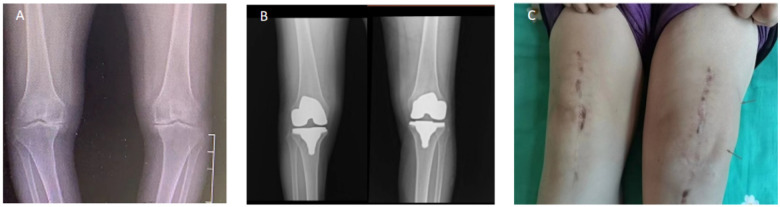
Radiographic and clinical presentation of the patient before and after total knee arthroplasty. (A) Preoperative standing anteroposterior radiograph showing severe bilateral knee osteoarthritis with varus deformity. (B) Postoperative radiograph after bilateral total knee arthroplasty demonstrating appropriate prosthesis alignment and positioning. (C) Clinical image showing significant swelling of the left knee approximately 11 months after surgery.
